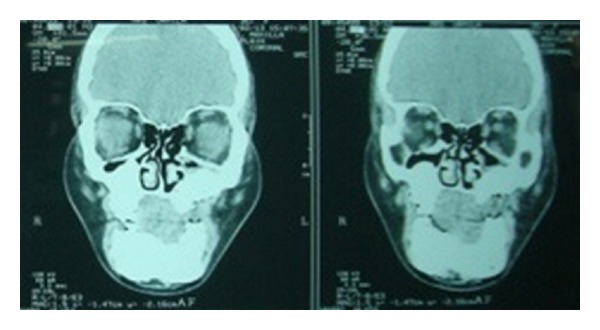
CT scan of patient's mother (Case 2).
